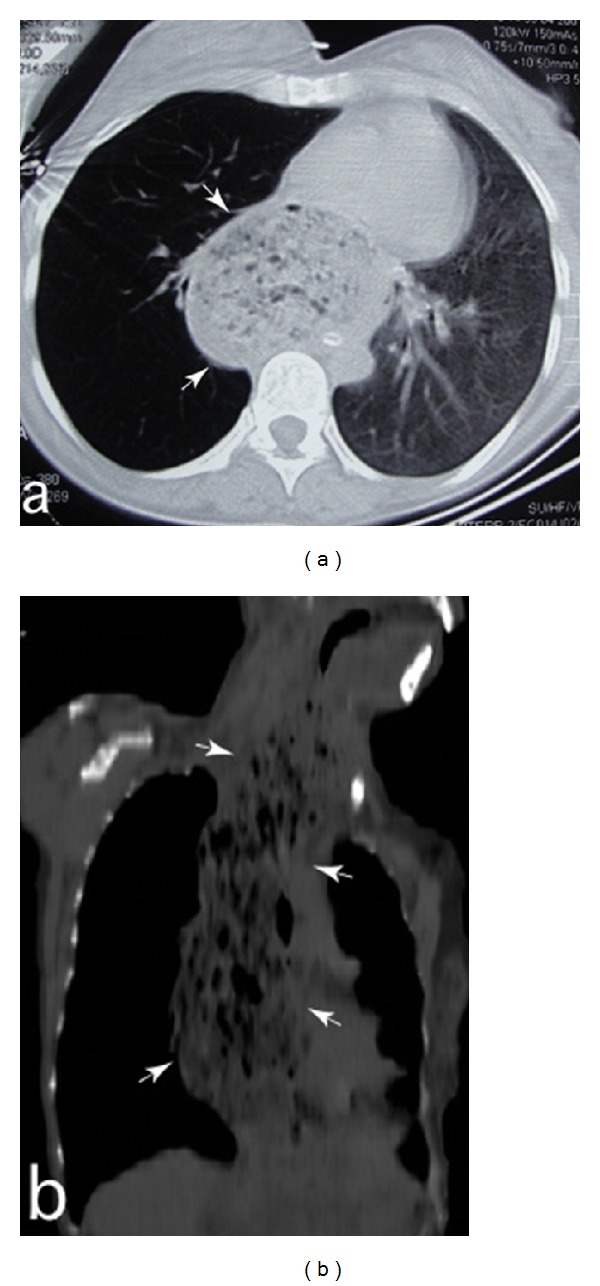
Thoracic computed tomography examination after intubation. (a) The axial cross-section shows a severely dilated oesophagus filled with food residue behind the trachea (arrows). The left lung parenchyma was infiltrative due to aspiration, and the heart was compressed by the dilated esophagus. (b) The coronal cross-section shows that the oesophagus is filled with food and air up to the cervical region (arrows).
